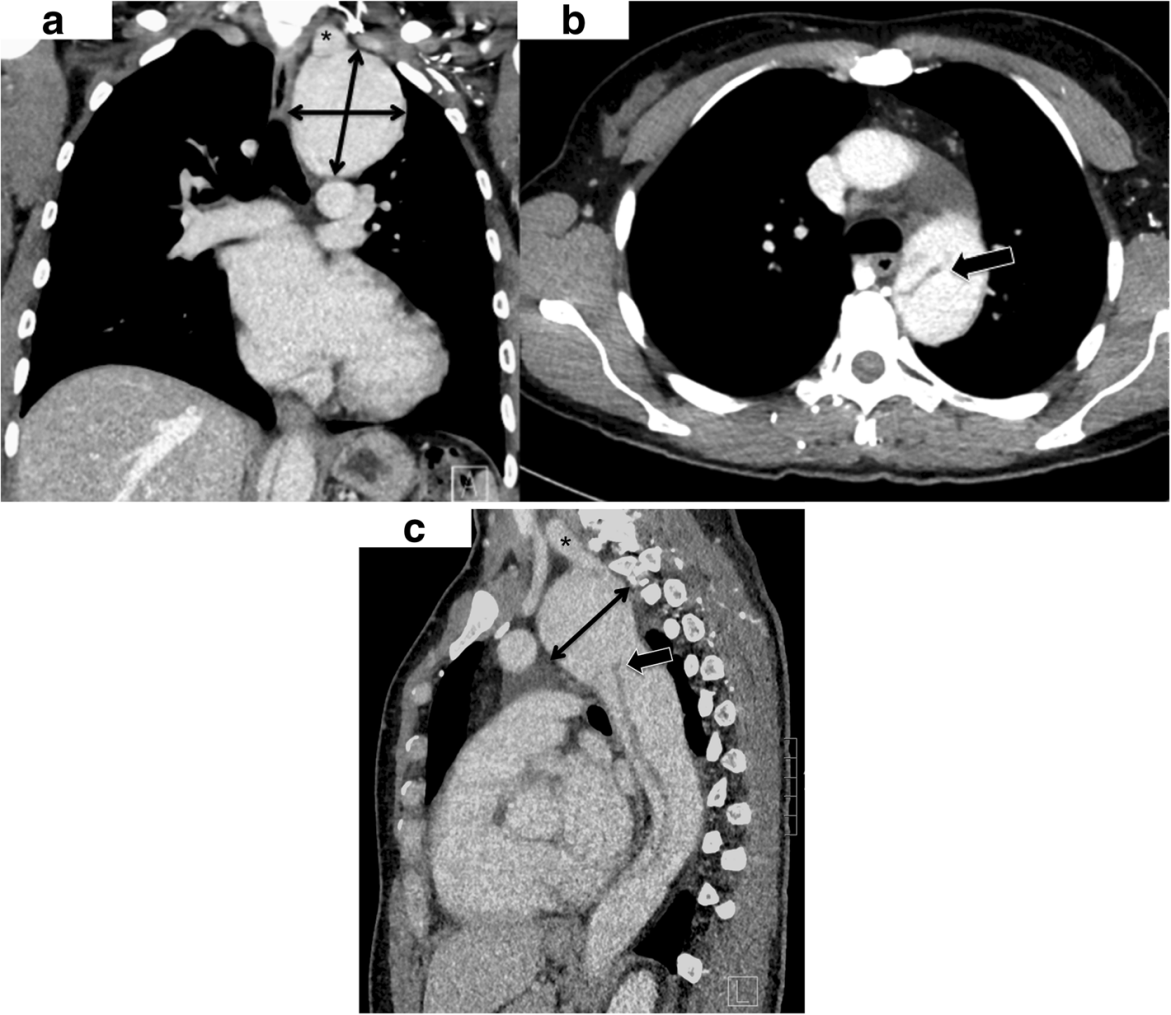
a Computed tomography of the cervical arch aneurysm with a maximal extend of 6 cm. * marks the origin of the left axillary artery. b Membrane of the type B dissection directly deriving from the aneurysm (arrow). c Lateral view with the localisation of the aneurysm in the upper posterior mediastinum. The subclavian artery branches from the top of the aneurysm and runs to the anterior part of the upper mediastinum (*). The dissection membrane starts inside the aneurysm to the abdominal aorta
